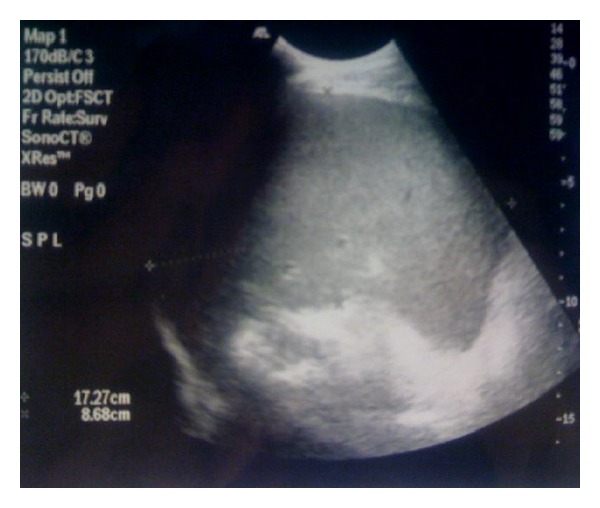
Abdominal ultrasound showed splenomegaly (17,27 × 8,7 cm) and free fluid in the left iliac fossa.
